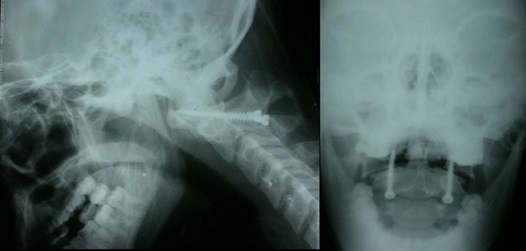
Post operative standard X-ray showing a patient who underwent transarticular screwing
